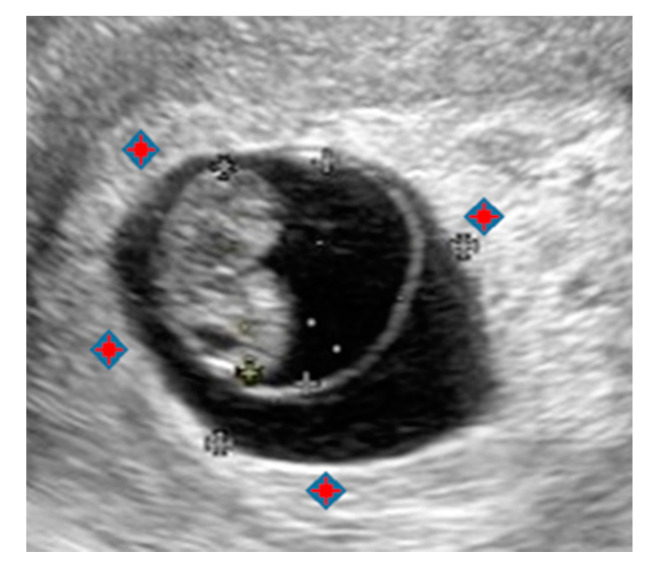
Ultrasonographic image at 7 w 3 d (19.3 × 13.8 mm). The red marks show the lacunar space where AE is injected.
